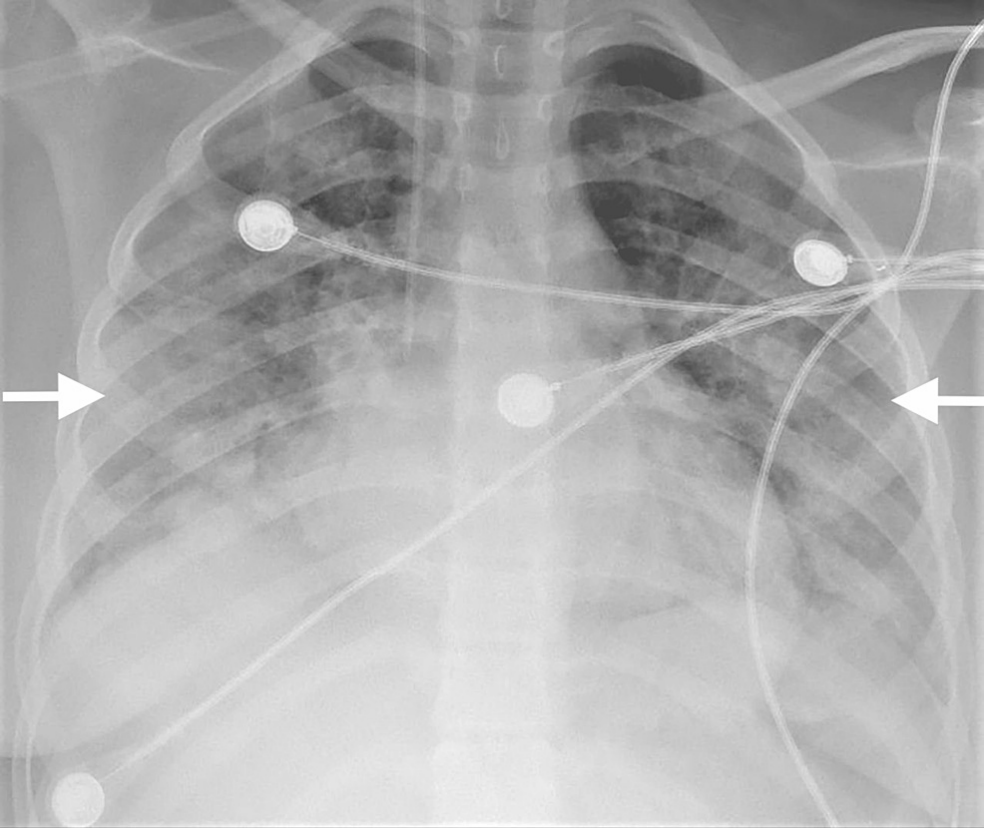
Chest x-ray showing bilateral pulmonary infiltrates.
